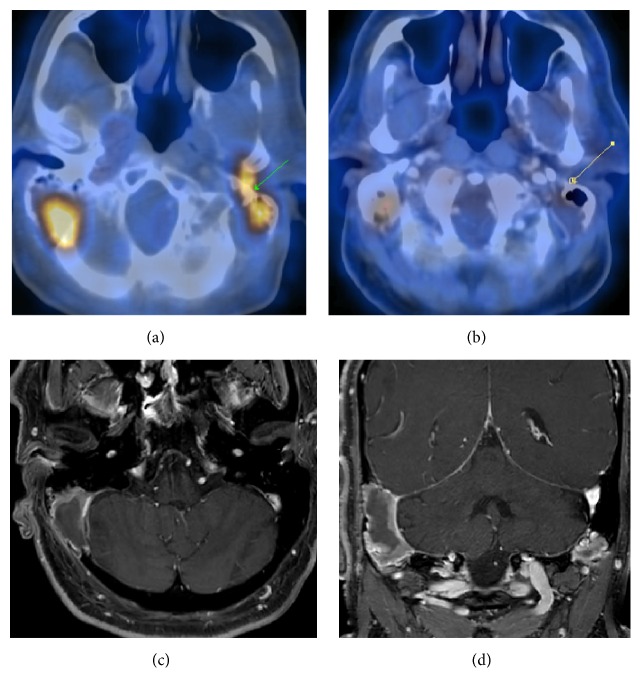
Posttherapy PET-CT scan (a, b) showing significant resolution in the metabolic activity of the mastoid lesions. Contrast enhanced MRI scan (c, d) also showed regression in the size of the enhancing soft tissue masses on both sides.
